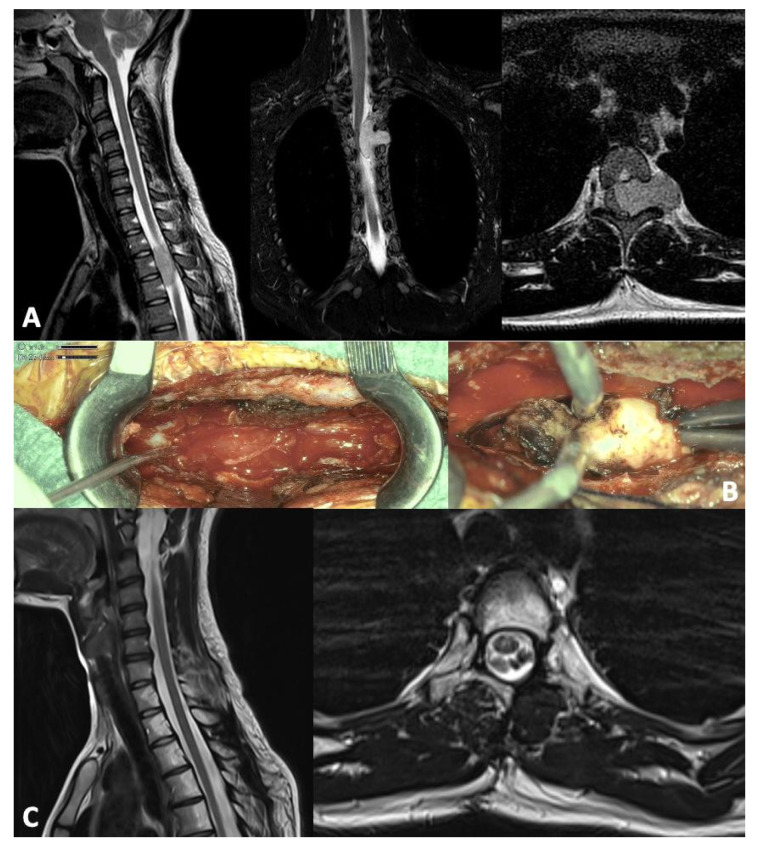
Preoperative T2-weighted MRI showing intradural extramedullary lesion with compression and dislocation of the spinal cord at level D3–D4 and left foraminal extension (A). Intraoperative views after left-sided laminectomy, appearance of solid fibrous mass at level D3–D4 with high blood supply (C). T2-weighted MRI at 2 years follow-up with no sign of relapse (B).
